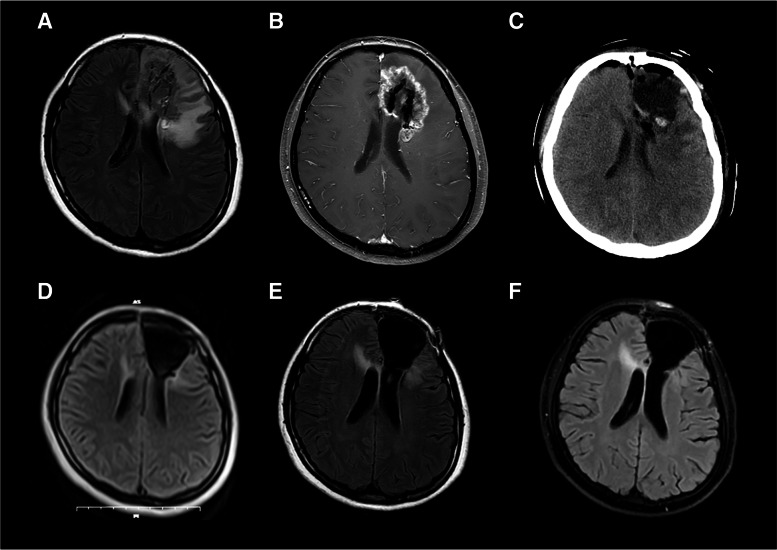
Routine imaging examinations of the patients. A T2WI-Flair image of the patient on 2018.07.27. B T1WI-contrast image of the patient on Dec.16, 2018. C CT image of the patient on January 27, 2019 after the surgical resection. D T2WI-Flair image of the patient on Apr.22, 2019. E T2WI-Flair image of the patient on May 08, 2019. F T2WI-Flair image of the patient on June 23, 2019
